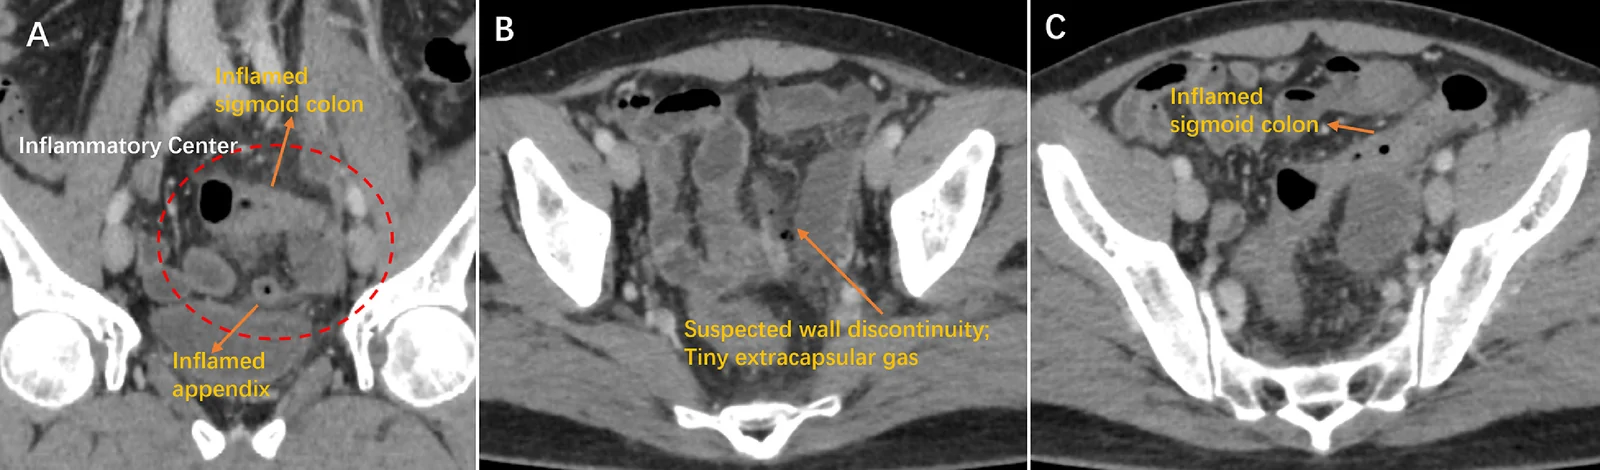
CT findings favoring left-sided appendicitis with suspected microperforation over primary sigmoid perforation. (A) Axial contrast-enhanced CT image demonstrates a dilated, thick-walled appendix with surrounding inflammatory change adjacent to the sigmoid colon. (B) Axial image demonstrates a suspected focal discontinuity of the appendiceal wall and tiny extraluminal air foci immediately adjacent to the suspected appendiceal wall discontinuity. (C) The adjacent sigmoid colon shows mild focal wall thickening without a definite wall defect or inflamed diverticulum, favoring secondary reactive change.
